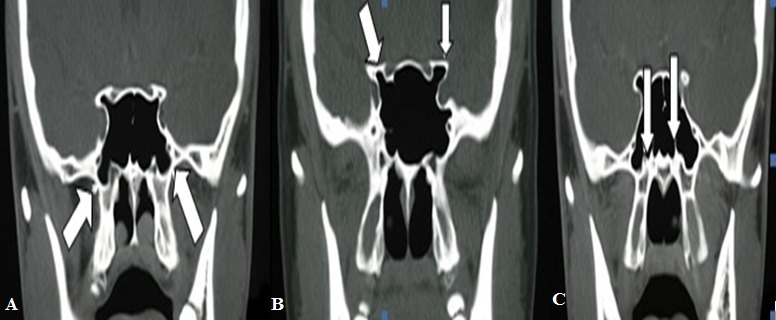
(A) bilateral Pterygoid process pneumatization in coronal PNS-CT; (B) bilateral anterior clinoid process pneumatization in coronal PNS-CT, (C) vidian canal protrusion in coronal PNS-CT
